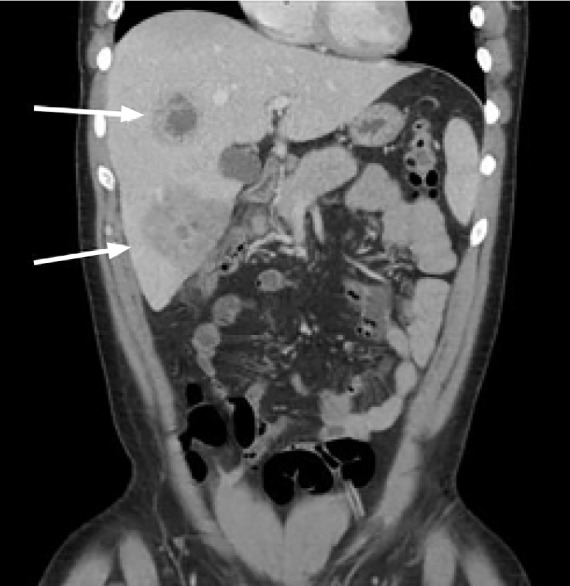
Coronal computed tomography of the abdomen and pelvis of 37-year-old male with intermittent fever and vague abdominal pain with hepatic abscess (arrows).
